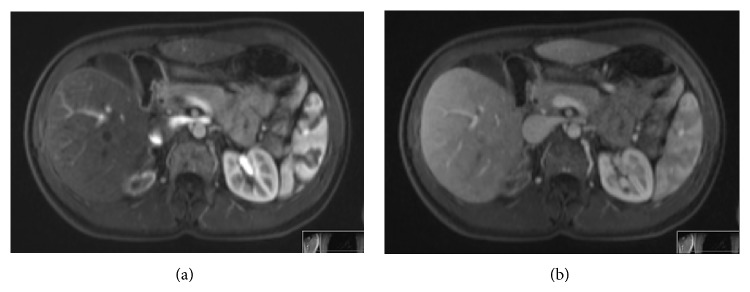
MRI axial T1-weighted images of the right kidney lesion after intravenous contrast medium administration. (a) Arterial phase, peripheral enhancement following contrast administration, and (b) venous phase.
